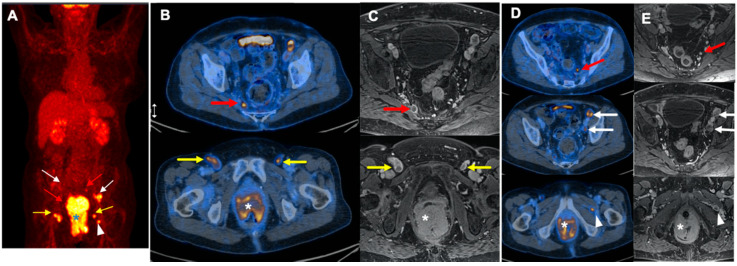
A 77-year-old female with T4N1cM0 disease. (A): MIP: maximum intensity projection and (B,D): (transverse fused PET/CT images): Aside from the primary tumor (*), there are multiple metabolically active lymph nodes in bilateral internal iliac (red arrows), external iliac (white arrows), and inguinal (yellow arrows) regions. Also, an intermuscular lymph node is visualized in near the left external obturator muscle (white arrow head). (C,E): MRI (magnetic resonance imaging), Axial T1 weighted flash3d vibe fatsat Sequence shows corresponding morphological changes (arrows).
